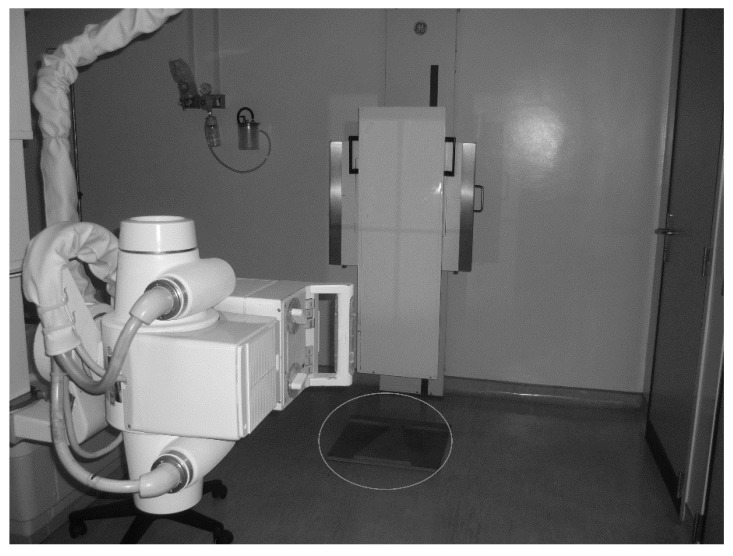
Philips X-ray machine with the wood base for the foot position.
